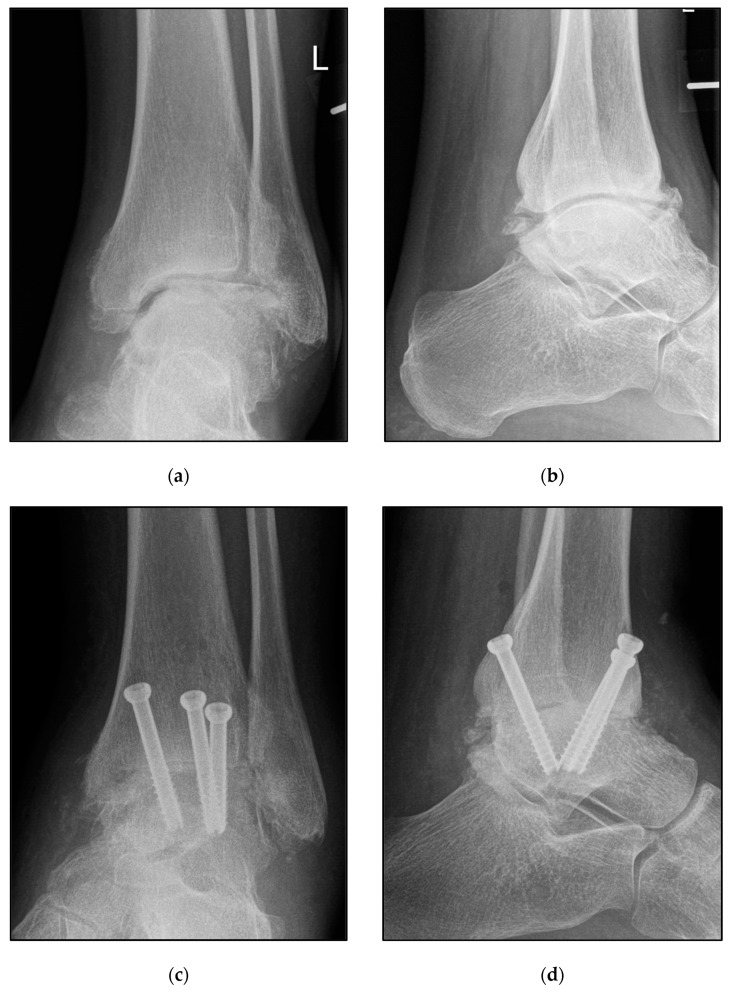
Pre- and postoperative radiographic findings of end-stage posttraumatic arthritis of the left ankle of a 44-year-old male treated with tibiotalar arthrodesis (TTA) using cannulated screws (diameter 6.5 mm). (a,b) Anteroposterior view, preoperative. (c,d) Anteroposterior view, 8 weeks postoperative.
